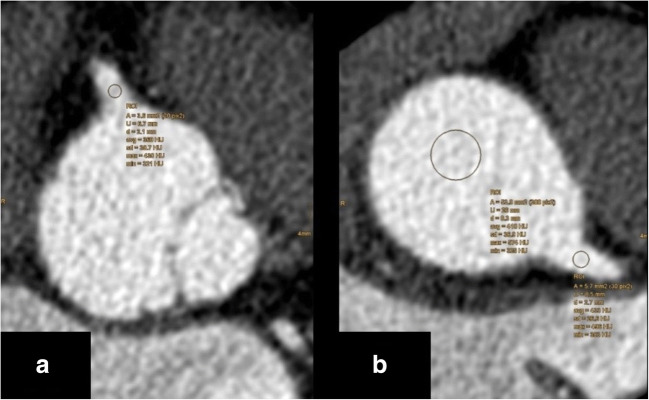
Positions of ROIs for quantitative analysis of coronary arteries (after completion of computed tomography angiography scan). a ROI in proximal right coronary artery; b ROIs in ascending aorta and left main coronary artery
